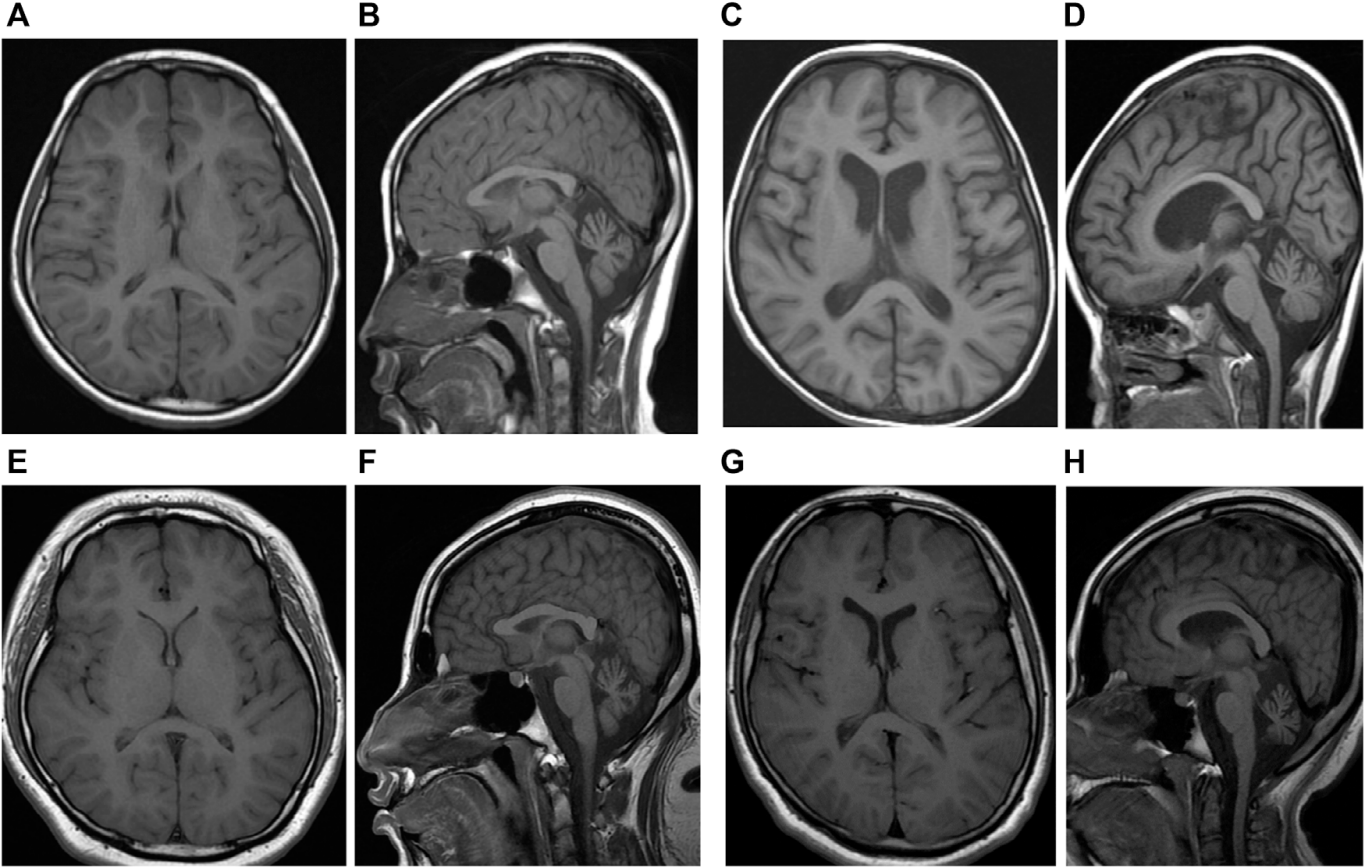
(A) T1-weighted axial and (B) sagittal image of sibling one performed at 9 years of age show diffuse cerebral, cerebellar and brain stem atrophy and relatively normal ventricle. (C)T1-weighted axial and (D) sagittal image of sibling one performed at 18 years were not changed significantly. (E)T1-weighted axial and (F) sagittal image of sibling two performed at 4 years of age show diffuse cerebral, cerebellar and brain stem atrophy and mild hydrocephalus. (G)T1-weighted axial and (H) sagittal image of sibling two performed at 16 years were not changed significantly.
